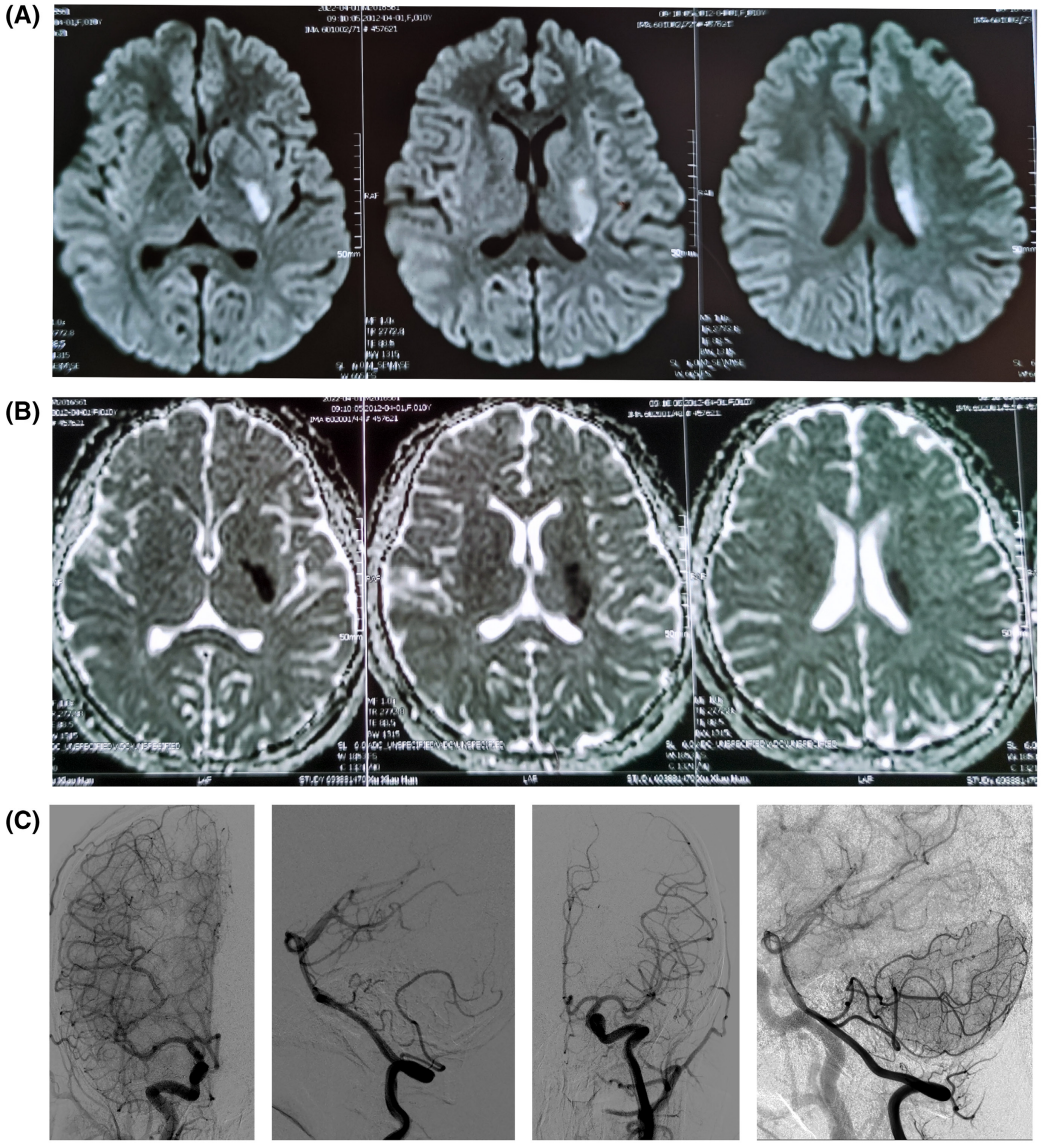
MRI and DSA images of this patient. Axial DWI (A) and ADC (B) images at the same level shows punctate foci of diffusion restriction in the left corona radiata and internal capsule. DSA show no vascular abnormality (C).
